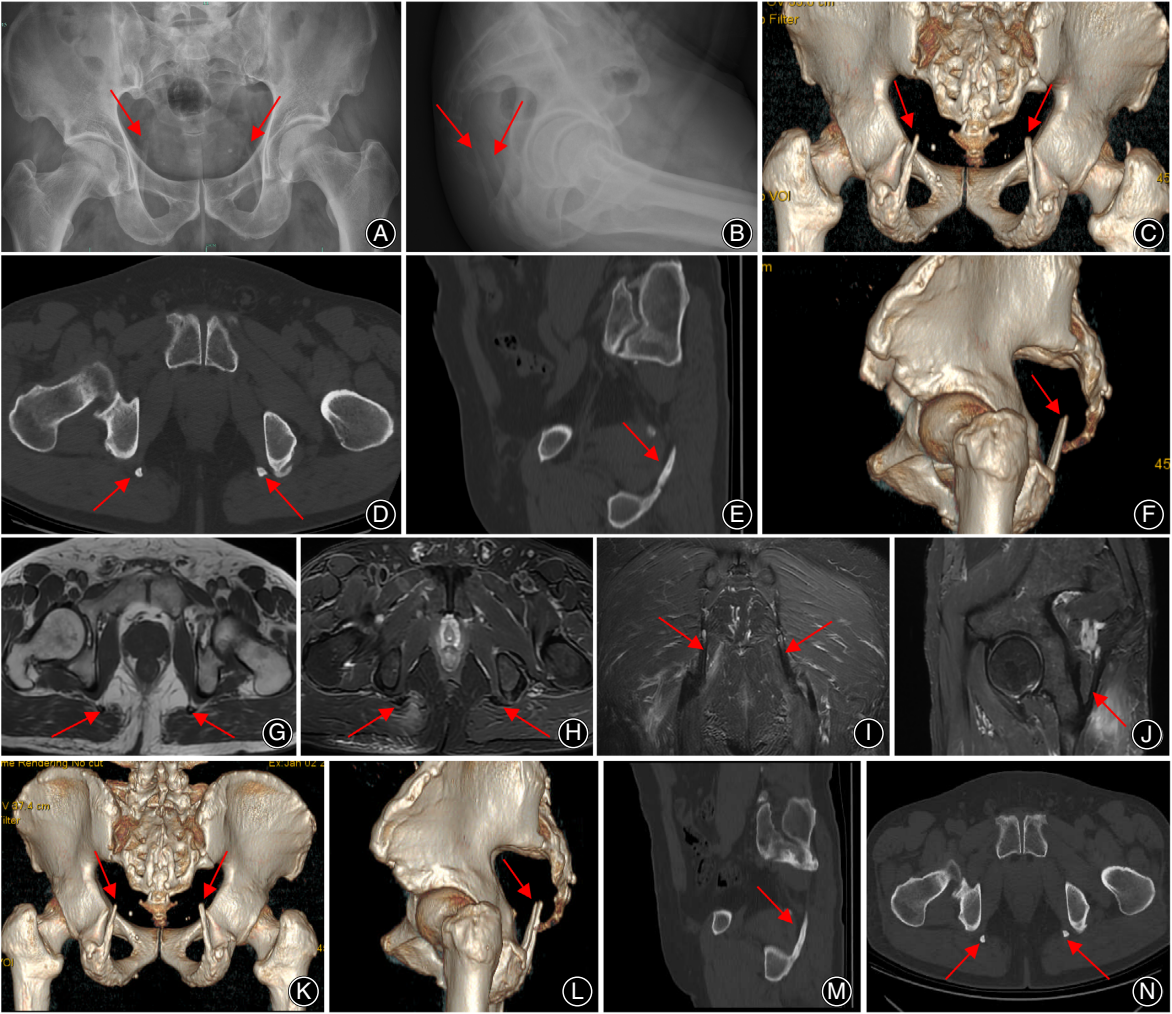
X‐ray, CT, and MRI images of the patient. (A, B) “Antelope's horns” sign on anteroposterior and lateral views of pelvis on x‐ray imaging. (C–F) CT showed a shape resembling fish bones. (G–J) MRI revealed on oblique sagittal (15 degrees) and cross‐sectional views of T1 and T2. (K–N) The follow‐up CT images taken after 12 months indicated that there were no noticeable changes in the ossification areas.
